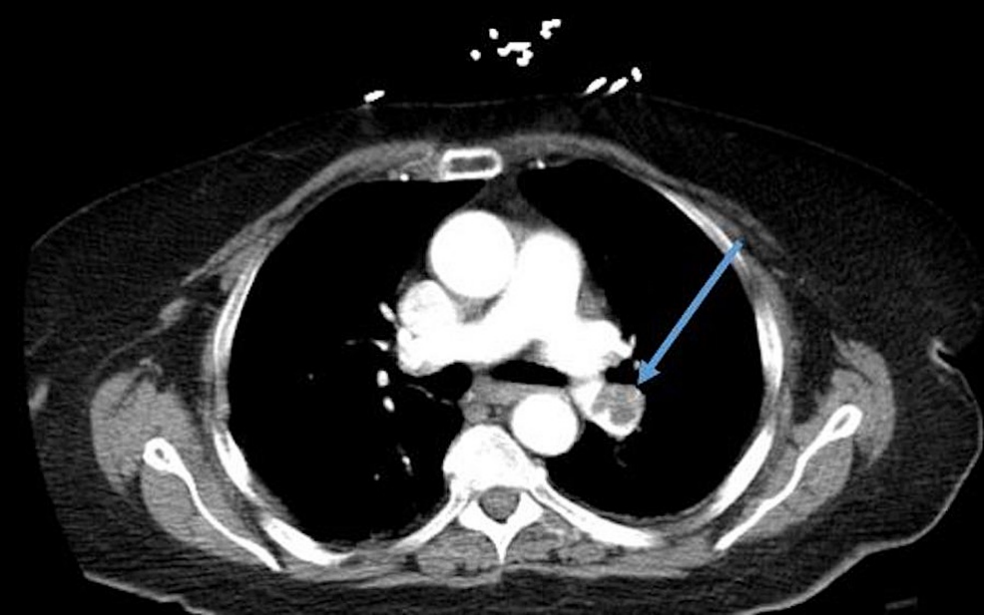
Computed tomography angiography (CTA) - blue arrow showing left pulmonary embolism
